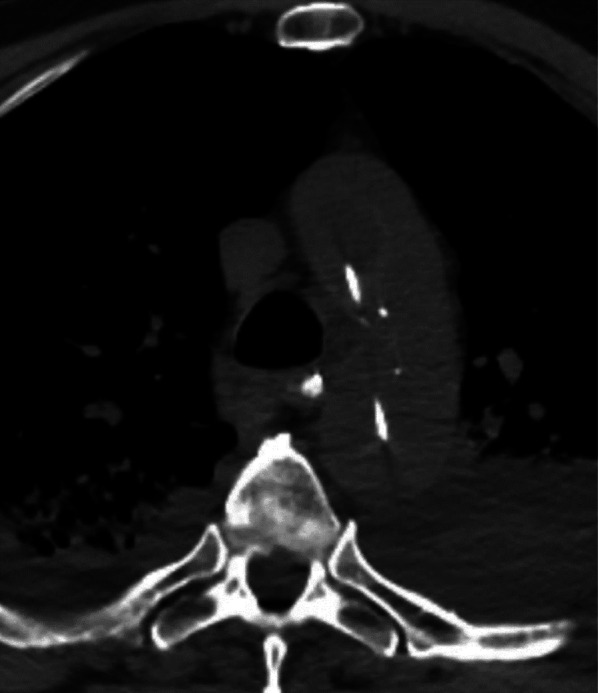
Complication of Impella device. Axial non-contrast CT in a patient on transfemoral Impella device demonstrates medial displacement of the intimal calcification. This is consistent with an acute aortic dissection
